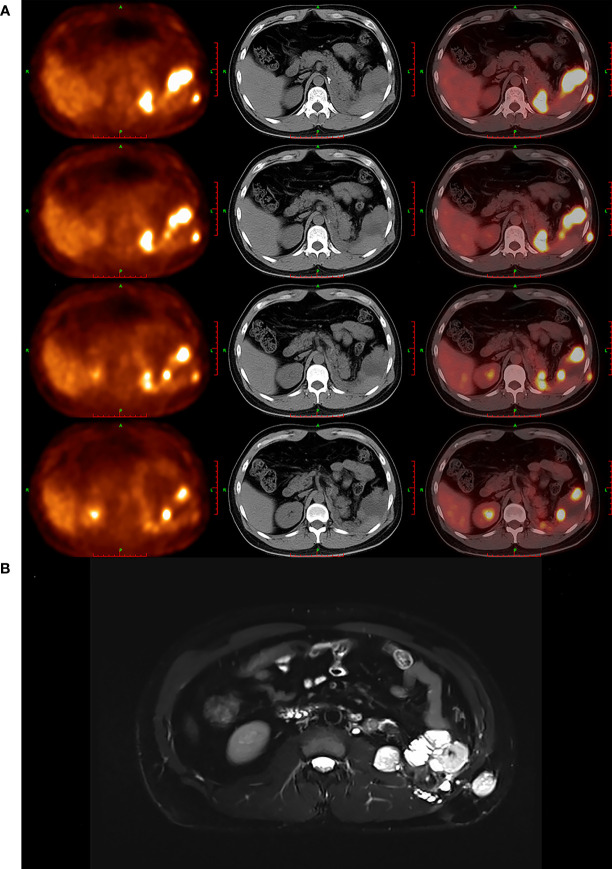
PET/CT and MRI findings of masses. (A) PET/CT images showed that multiple soft-tissue-density nodules and masses were found in left renal and adrenal region, perisplenic region, left retroperitoneum, left intraabdominal fascia, and left abdominal wall, which featured high uptake of ^18^F-FDG abnormally. (B) MRI images showed that multiple nodules and masses were found in left renal and adrenal regions, perisplenic region, left retroperitoneum, left vertebral fascia, subcutaneous and muscular layer of left posterior abdominal wall, anterior part of left psoas major muscle, and left iliac fossa, which suggested that the tumor has recurred and metastasized likely.
